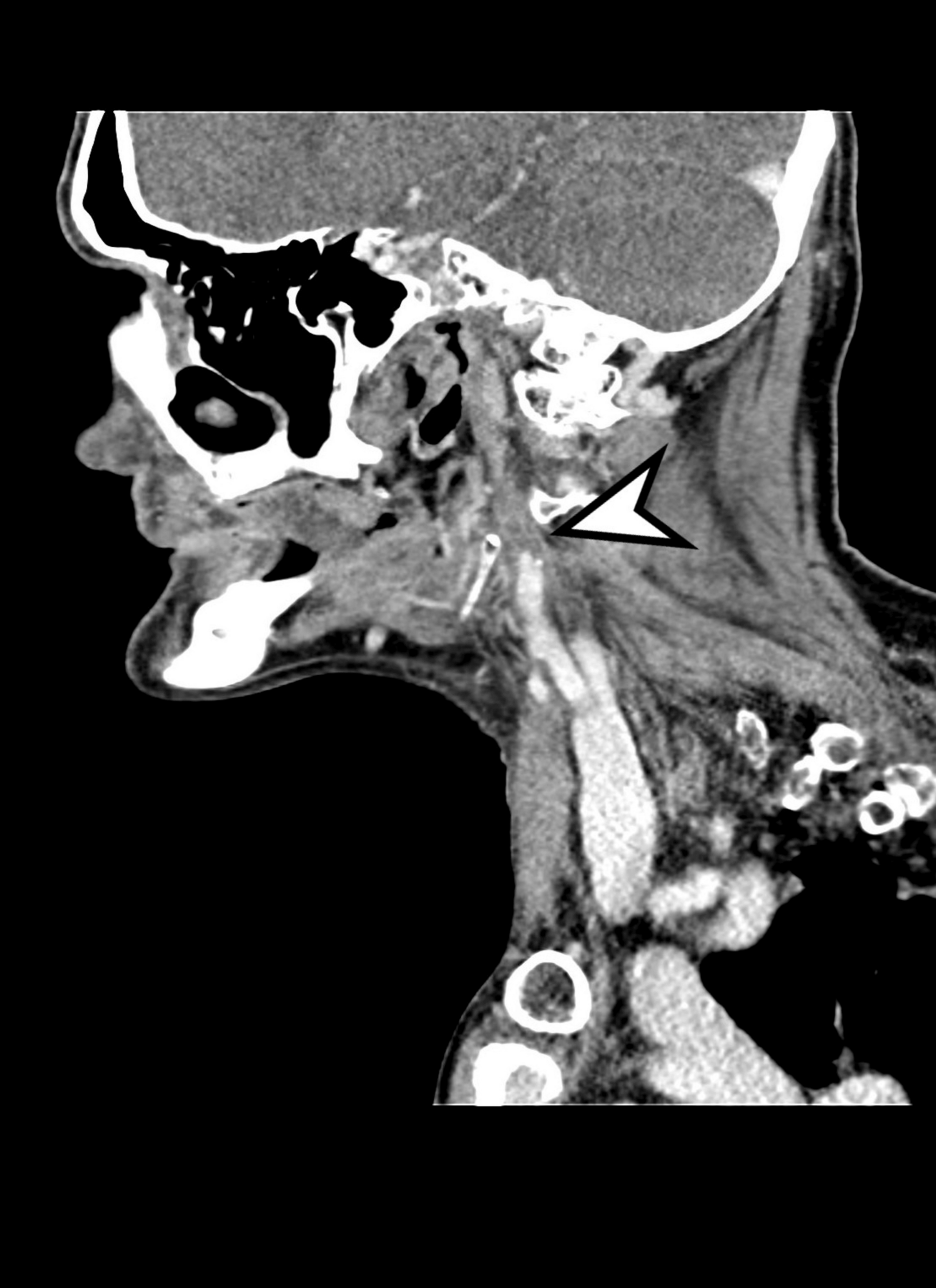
Sagittal reconstruction image from computed tomography angiography (CTA) demonstrating complete occlusion of the right cervical internal carotid artery (white arrowhead).
